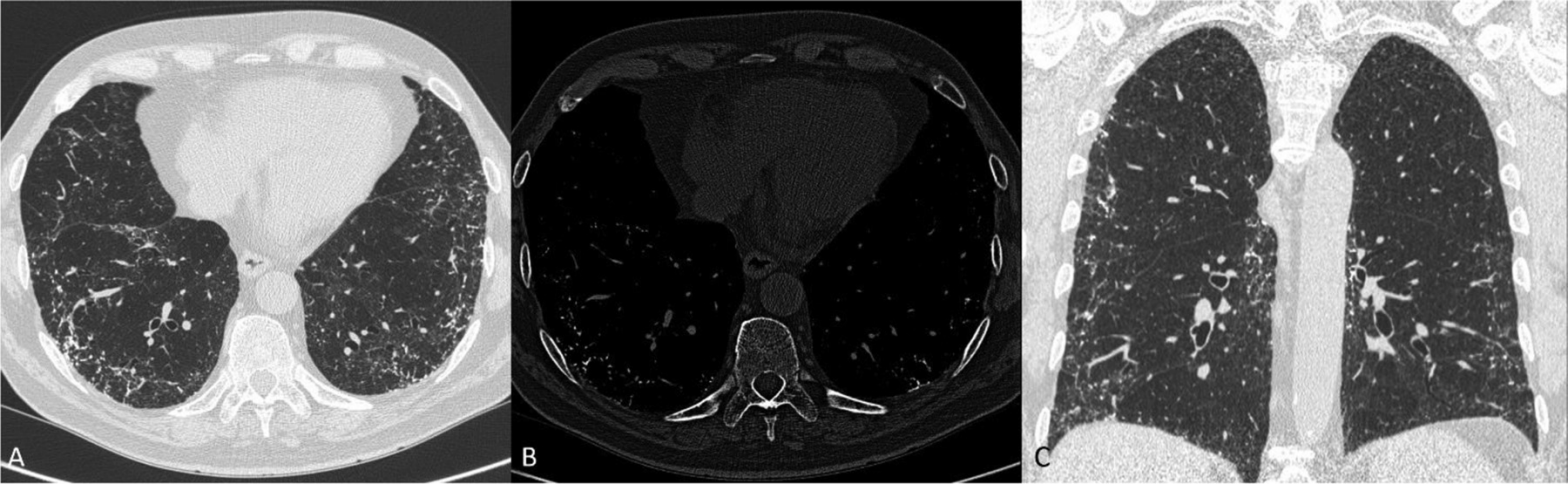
A - B - C. High resolution computed tomography (HRCT) scan, lung (A) and bone window (B), showing small, calcified nodules and branching opacities at lung bases, in absence of signs of lung fibrosis. Coronal reconstructed image depicts the cranio-caudal distribution of the abnormalities.
